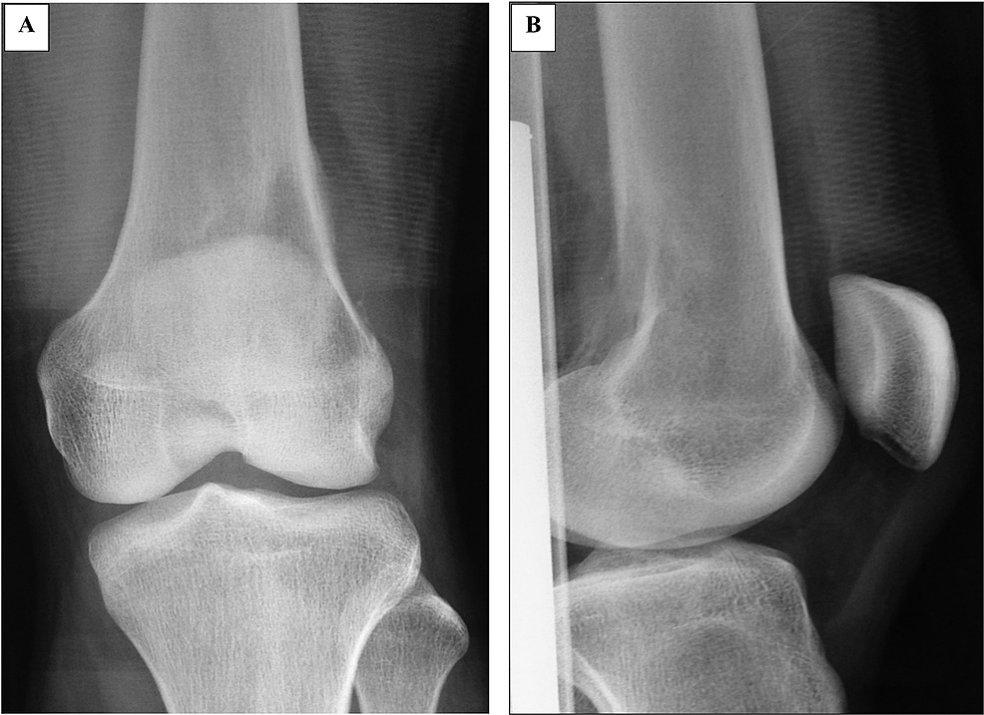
Plain radiographs of the right distal femur before the initial surgery Retrospective review of the anteroposterior (A) and lateral view (B) images revealed the presence of an ill-defined osteolytic lesion in the distal femoral metaphysis with minimal soft tissue opacity at its posterolateral aspect. The articular surface and space of the knee joint were preserved. These features were in keeping with an aggressive lesion involving the distal femur.
